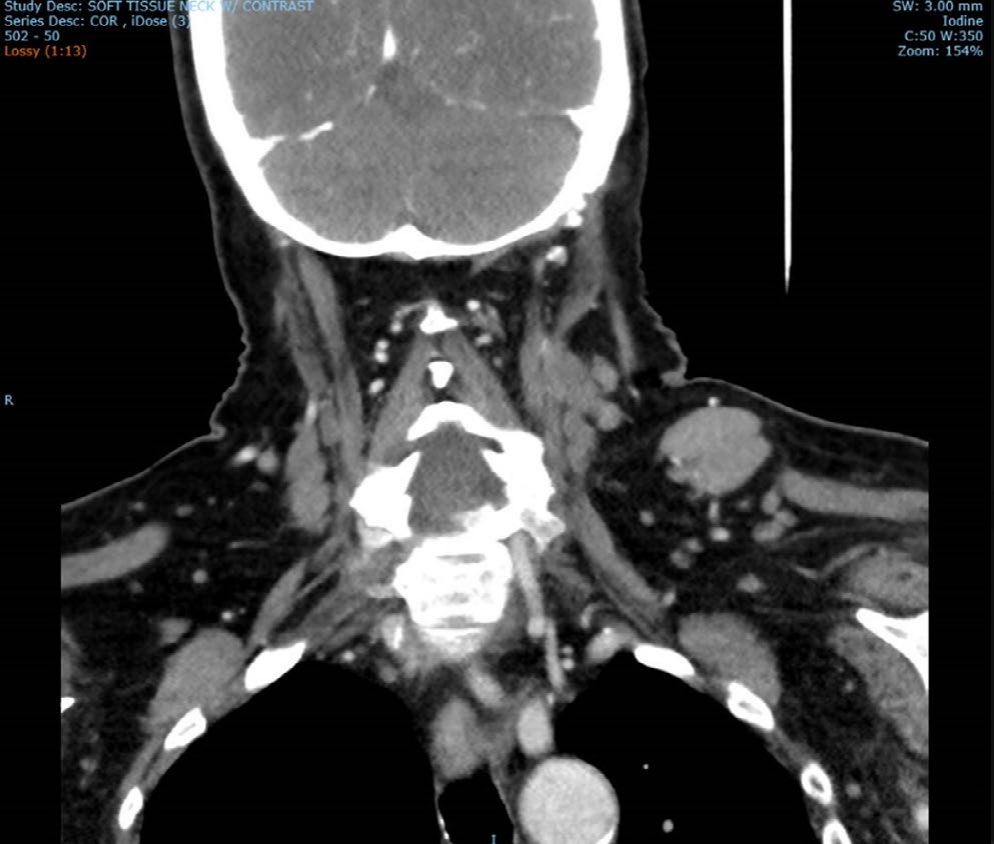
Coronal CT soft tissue Virchow's node
